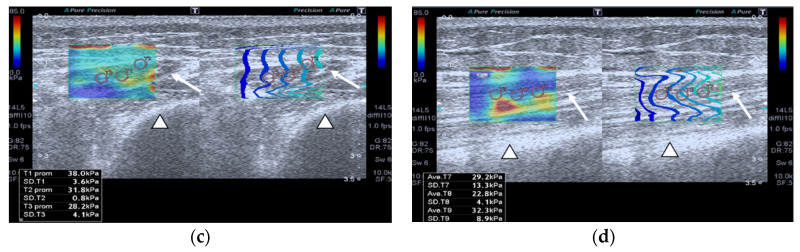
Quadriceps rectus femoris (QRF) muscle ultrasound (US) scan and shear wave elastography (SWE) with several regions of interest (ROIs) (pink circles) measured across the muscle of a matched healthy control (63 years old). SWE shows high muscle elasticity. (a) Transversal QRF muscle (arrow) US scan, femur (arrowhead); (b) longitudinal QRF muscle (arrow) US scan, femur (arrowhead). Patient with multiorgan failure (67 years old). SWE shows muscle stiffness with several regions of interest (ROIs) (pink circles). (c) Transversal QRF muscle (arrow) US scan, femur (arrowhead); (d) longitudinal QRF muscle (arrow) US scan, femur (arrowhead).
